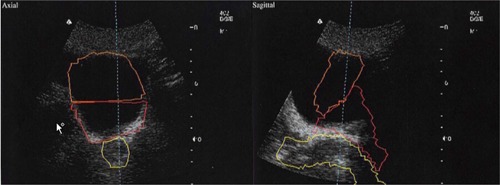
BAT template alignment. The BAT system is forced to align the cross sections of the volumes (rectum, bladder, and prostate bed) to the ultrasound image according to the CT shifts on the particular day.
